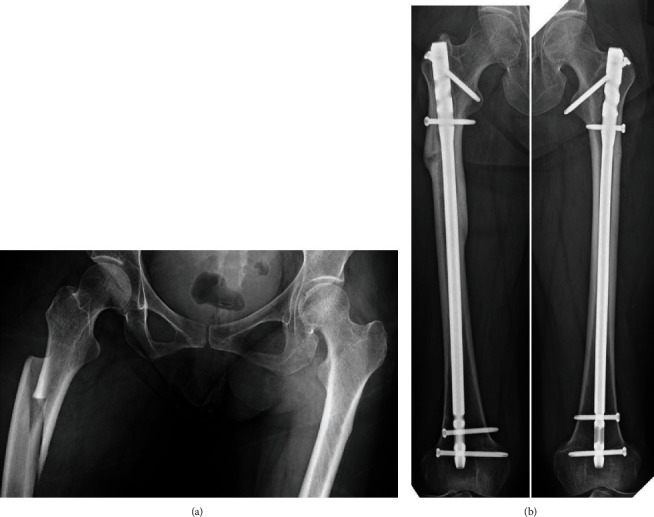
Both femur roentgenograms of case 4 at the time of injury (a) and final follow-up (b). There were no curvatures at both femoral diaphyses.
